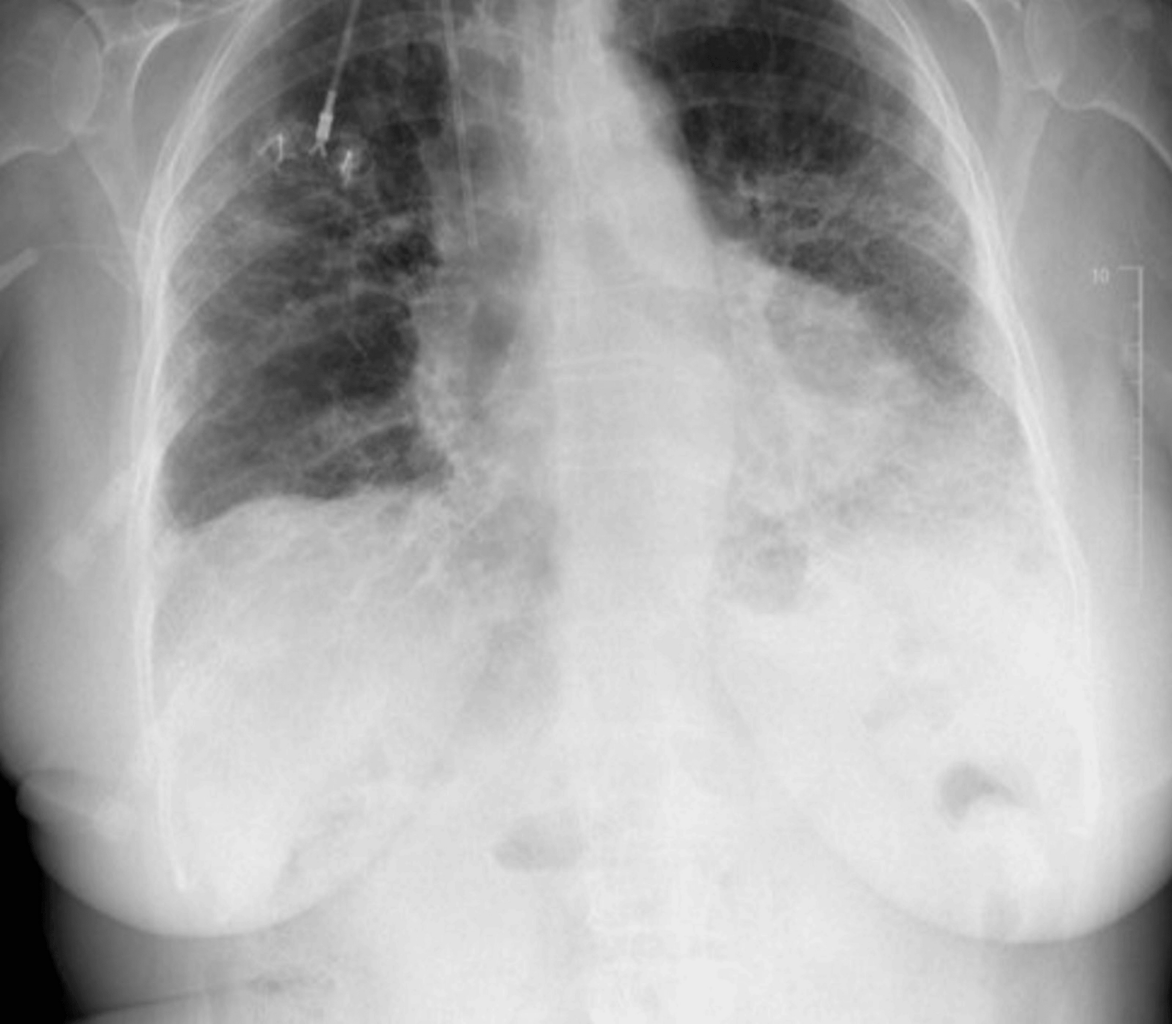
Chest radiograph demonstrating bilateral reticulonodular opacities and patchy consolidation consistent with chronic interstitial lung disease related to GPA. GPA: granulomatosis with polyangiitis
